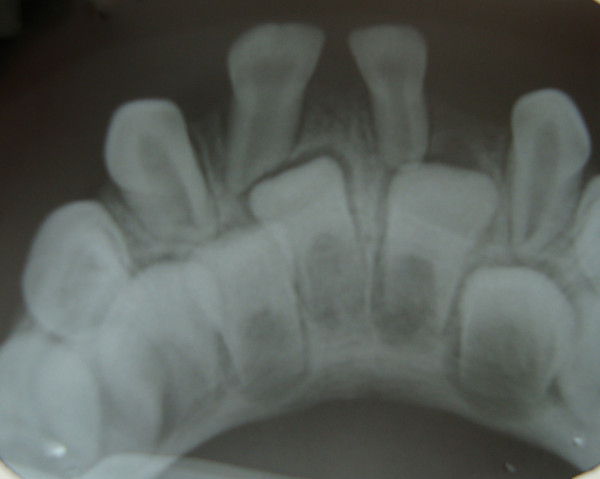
Periapical radiograph displaying a geminated mandibular right central primary incisor with one root and one root canal and absence of a mandibular permanent left incisor.
